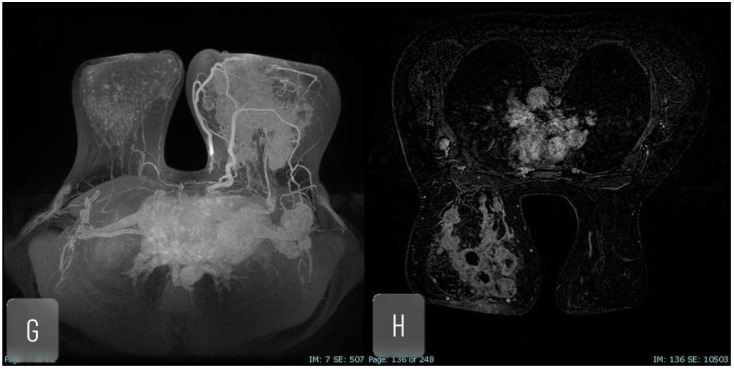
Forty-two yo. breastfeeding woman, with swollen left breast, not responding to antibiotics. Prior colorectal cancer treated with surgery and chemotherapy (Patient with known Lynch Syndrome). Mammography detected a large radiopaque area (70 mm) with calcifications. A core needle biopsy was performed, which detected high grade ductal infiltrant carcinoma associated with DCIS component (high nuclear grade). In homolateral axillary cave lymphadenopathy was detected (cytological examination revealed lymphonodal involvement). A MRI was performed with a dimensional upgrade of the lesion. (A) MLO mammographic projection; (B) CC mammographic progression; (C,D) US imaging, breast lesion; (E,F) US imaging, axillary lymph node; (G,H) MRI, MIP and contrast enhanced imaging.
